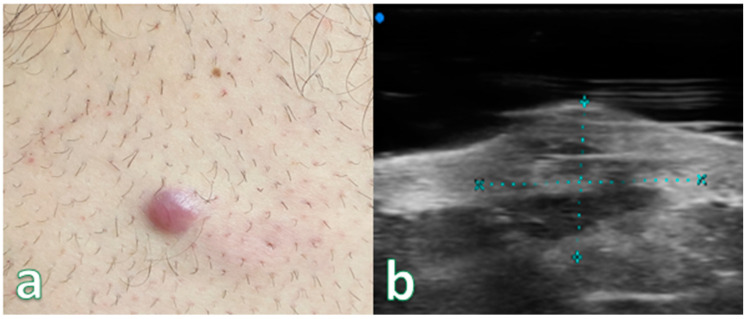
(a) Reddish nodular skin lesion without signs of flogisis in the anterior region of the right leg. (b) Examination performed with a very high-frequency ultrasound probe at 50 MHz showing iso-hypoechoic lesion with irregular and hyperechoic margins infiltrating the epidermis and the subepidermal layer highlighted with dashed blue line. Final pathologic assessment revealed to be a B-Cell Lymphoma.
